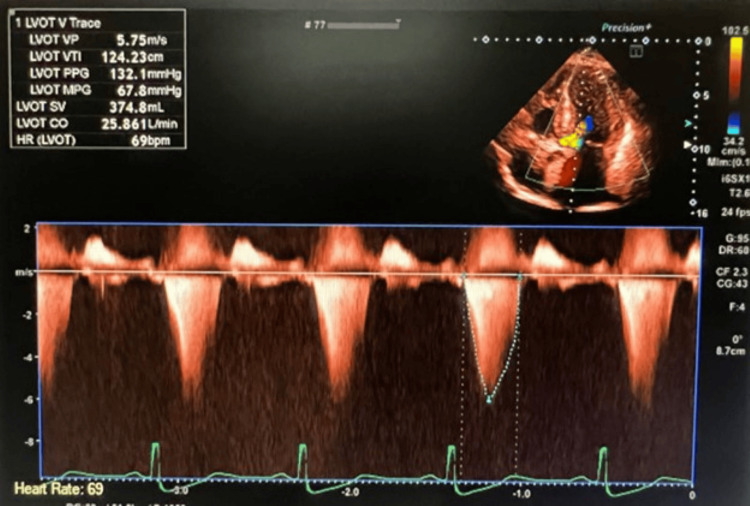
Transthoracic echocardiogram with a pulsed wave Doppler of the LVOT in a five-chamber view pre-ventriculomyotomy-myectomy showed a resting peak gradient of 132.1 mmHg, which was significantly elevated.
